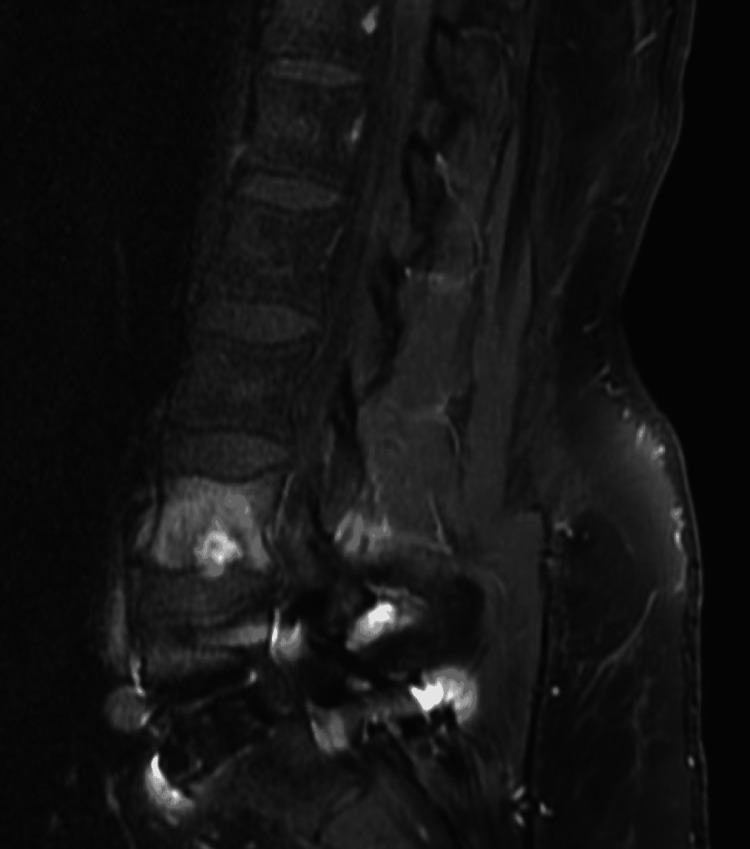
Sagittal MRI short tau inversion recovery sequence with lumbar 4 Schmorl's node.
